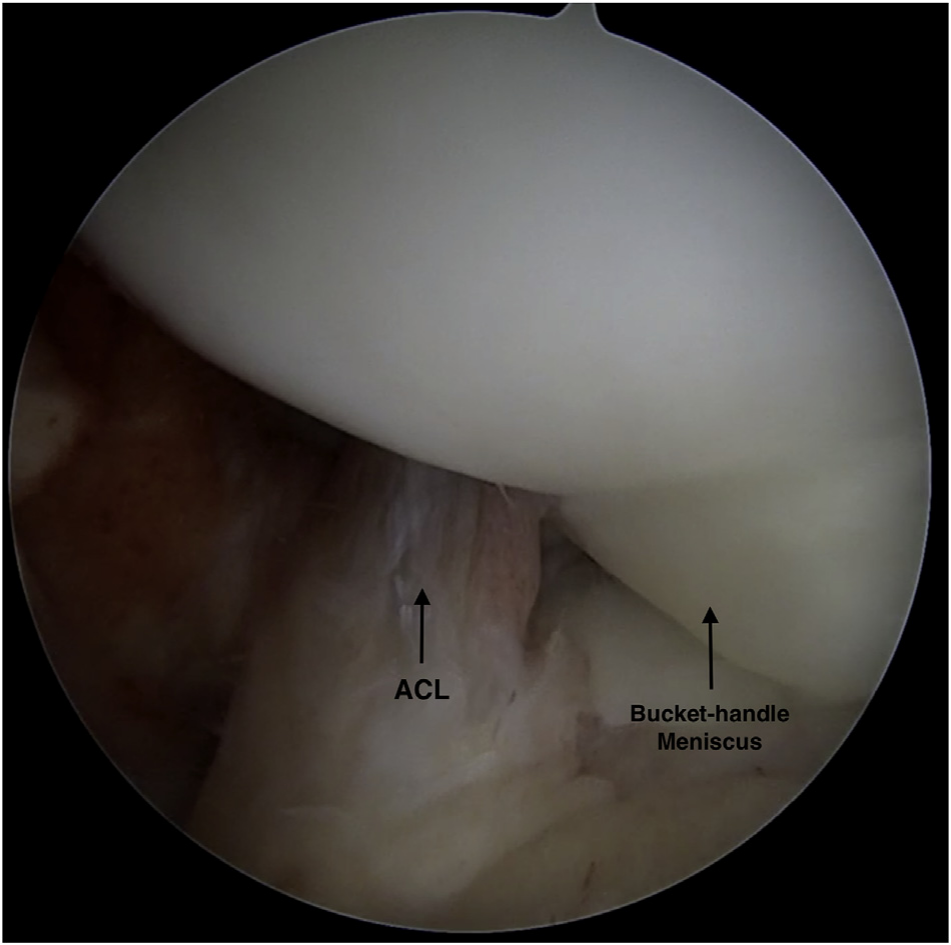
MRI of left knee. A) T2 weighted coronal view shows a meniscal fragment within the intercondylar notch. B) T2 sagittal view shows vertical tear in the peripheral zone of lateral meniscus. C) T2 sagittal view with an arrow points to the meniscal fragment entrapped behind ACL forming the double ACL sign. D) T1 weighted coronal view shows a meniscal fragment parallel to ACL within the notch.
